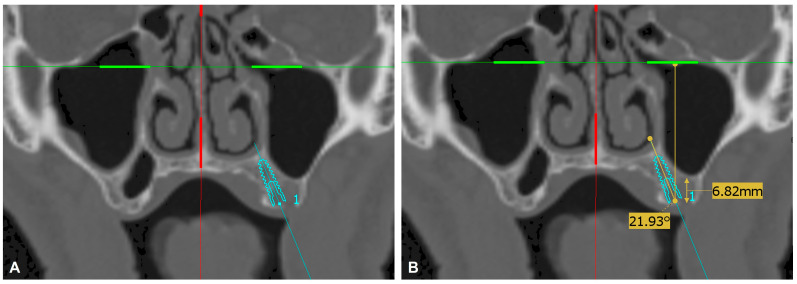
Helical CT coronal view. (A) An ICE implant 3.75/13 mm is virtually placed at the position of the first left maxillary molar, not penetrating the cortical borders. (B) The long axis angle of the implant is less than 30°, and the height of the alveolar crest is less than 8 mm.
